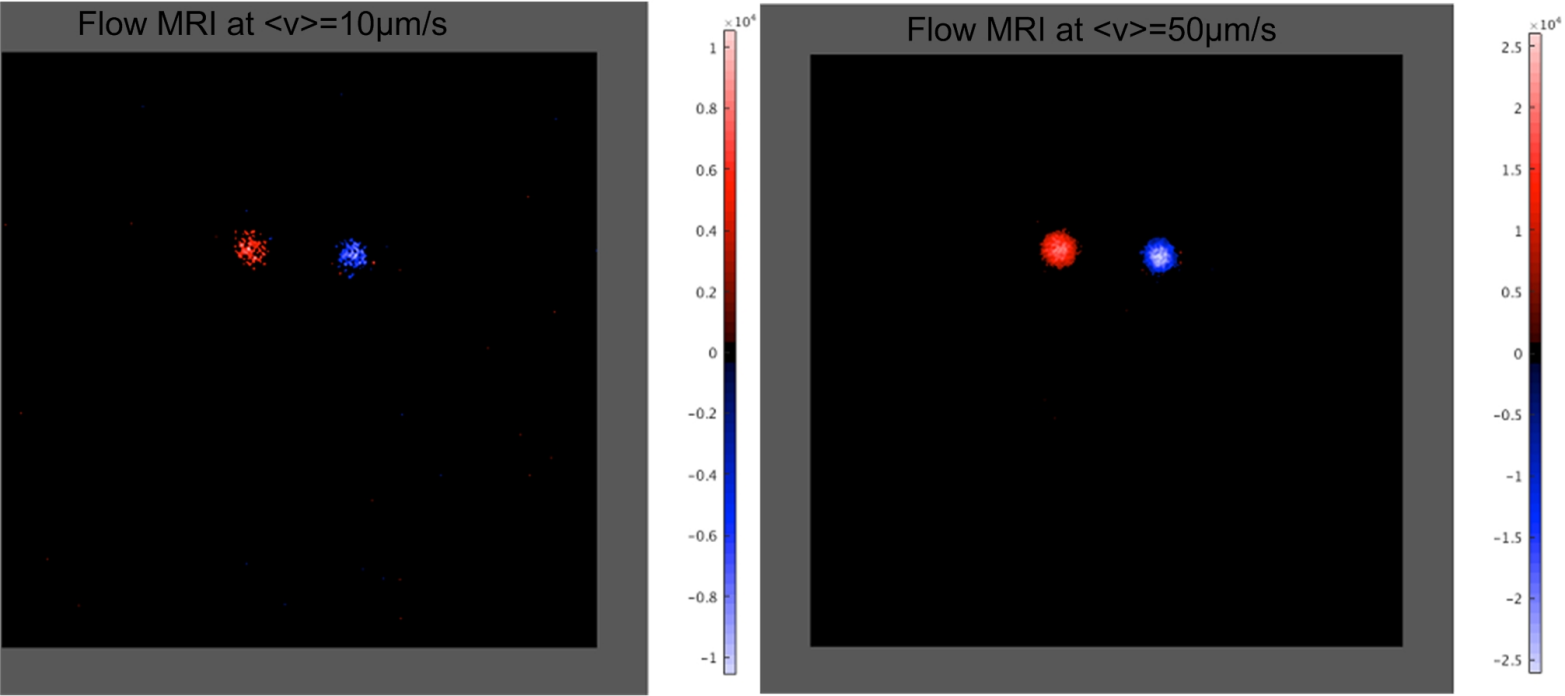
Flip-flop flow-MRI. Right figure: at 10*μm*/*s* and left figure: 50*μm*/*s*. The following parameters were used: *T*_*R*_ = 1.2*s*, *T*_*E*_ = 15*ms*, *FOV*_*READ*_ = 40*mm*, *FOV*_*PHASE*_ = 40*mm*, *N*_*READ*_ = *N*_*PHASE*_ = 256, *T*_*hk*_ = 0.5*mm*, 2 slices, *AVG* = 2 and *T*_*acq*_ = 2 × 10*min*14.
